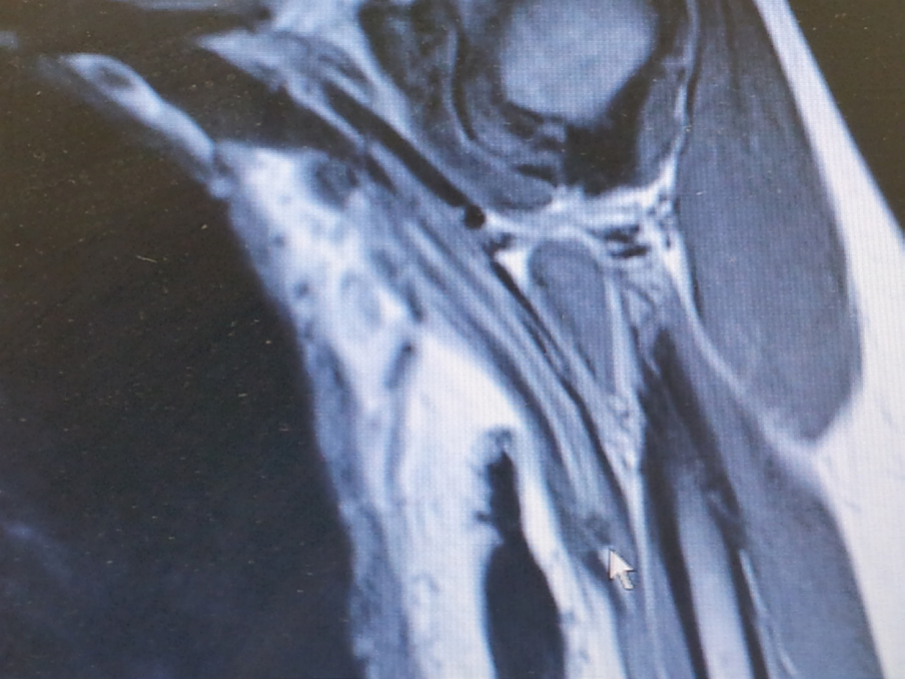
Magnetic resonance imaging of the median nerve showing a hypointense lesion of the nerve at the level of the midarm (arrow).
